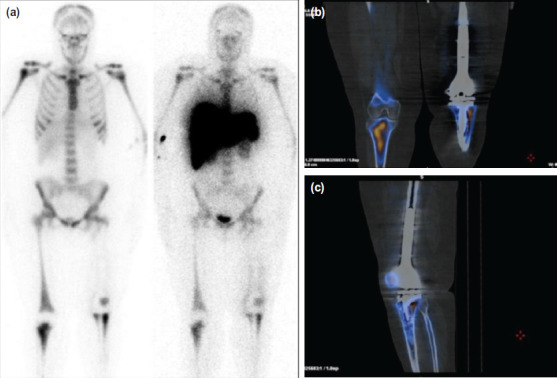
(a) Anterior view of whole-body scan with ^99m^Tc-besilesomab (left) and ^99m^Tc-sulfur colloid (right). Both images showed concordant radiotracer uptake at the left patella, and medial and lateral aspect of left proximal tibia prosthesis. No abnormal radiotracer accumulation was seen in the distal left femoral prosthesis, and tracer distribution elsewhere is physiological. Hybrid SPECT/CT image for ^99m^Tc-besilesomab in (b) coronal and (c) sagittal view. The addition of CT helps to delineate and localize the physiologic uptake pattern of bone marrow. No abnormal uptake were observed on the lower limb soft tissue.
